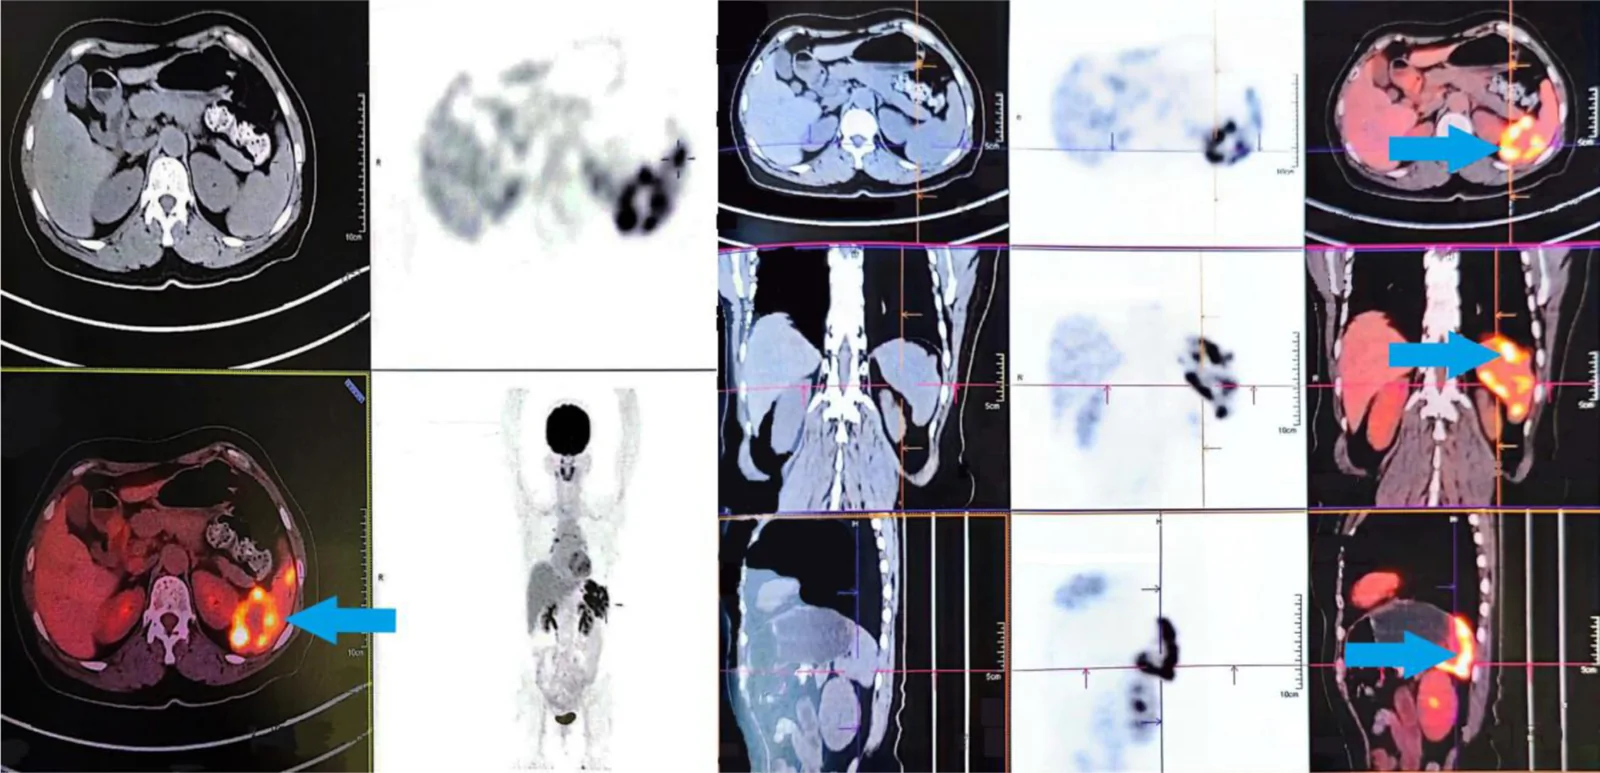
PET-CT demonstrating multiple hypermetabolic lesions in the spleen. PET-CT = positron emission tomography-computed tomography.
